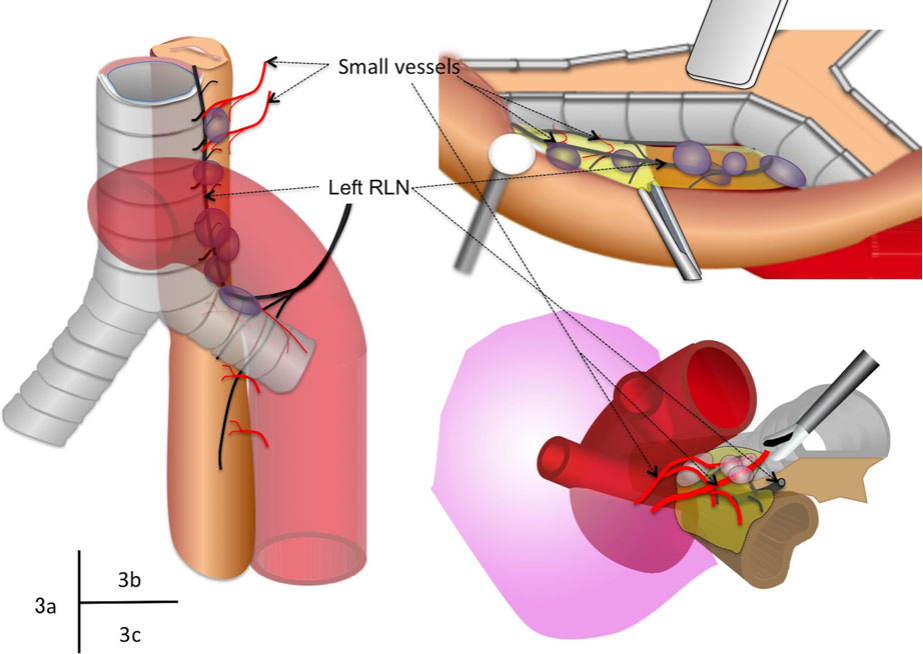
Location of the left paratracheal, left tracheobronchial nodes, adjacent vessels and nerve fibers. (A) Anterio‐left lateral oblique view. Left recurrent laryngeal nerve and lymph nodes are arranged on the opposite side from the right thoracic approach. (B) Surgical view of the right transthoracic approach. Skillful retractions of the trachea and the esophagus are required to expose the lymph nodes and the left recurrent laryngeal nerve. (C) Surgical view of video‐assisted transcervical mediastinal dissection (VATCMD). Lymph nodes were accessible without retractors and a bipolar sealer was placed in a suitable position to dissect small vessels and nerve branches.
